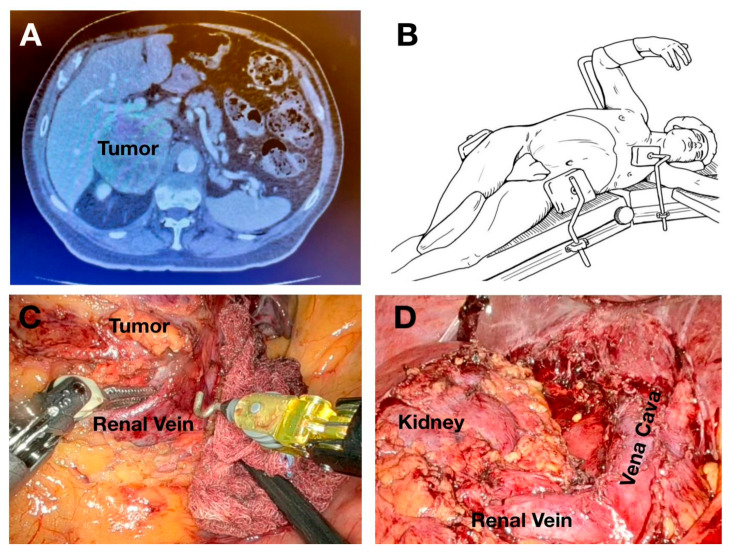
Right adrenal metastasis evidentiated on CT scan (A); patient position on the table for right robotic adrenalectomy (B); robotic dissection from surrounding structures (C), and final intraoperative view (D).
